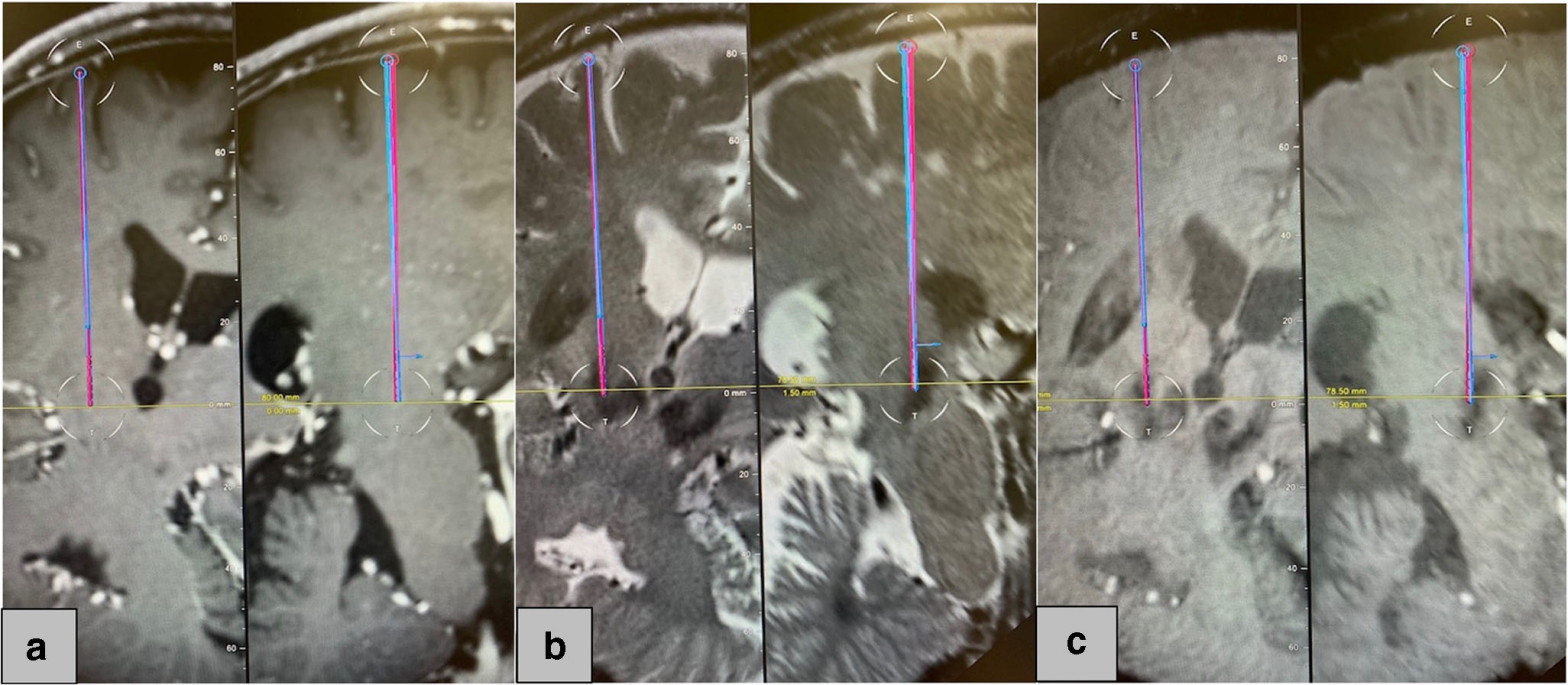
Postoperative lead localization and fusion (by means of the Brainlab® software Elements) with the preoperative MRI and the CT scan after the first lead implantation visualized on the T1 sequence after gadolinium (a), T2 sequence (b), and SWI (susceptibility weighted imaging) sequence (c). The initial lead position is shown as red trajectory and the lead position after the lead re-implantation as blue trajectory
